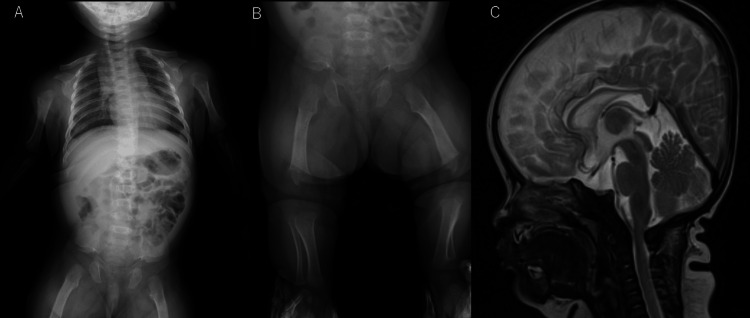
Clinical features of case 2. (A) X-ray image of the body at two months and 12 days of age showing short, robust tubular bones, squared-off iliac wings, flat acetabula, marked narrowing of the sacrosciatic notch, and a characteristic proximal femoral radiolucency. (B) X-ray image of the legs at two months and 12 days of age showing the same finding as in image A. (C) MRI at two months and 12 days of age showing mild foramen occipitalis magnum stenosis.
